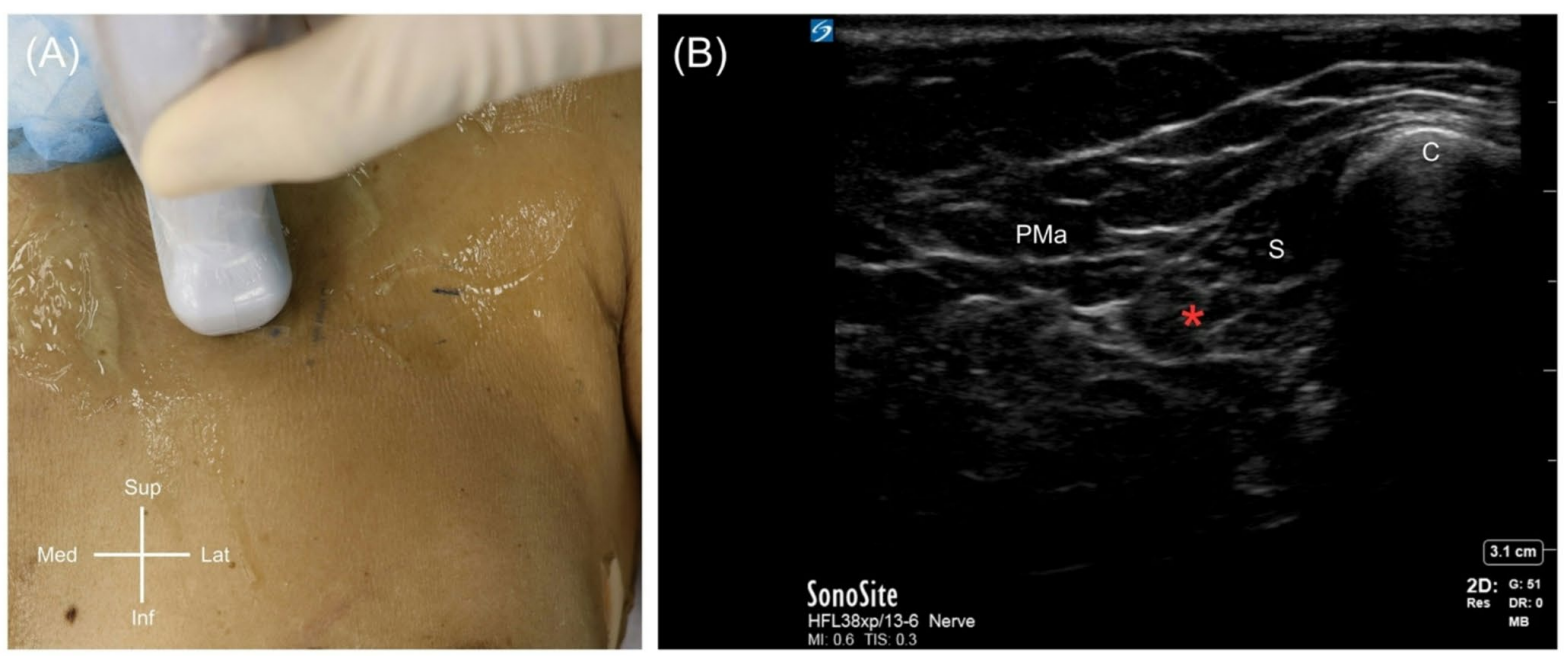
Probe placement for ultrasound examination (A) and corresponding high-resolution sonographic visualization (B) of the left subclavius posticus (red asterisk). Pma, pectoralis major; C, clavicle; S, subclavius
